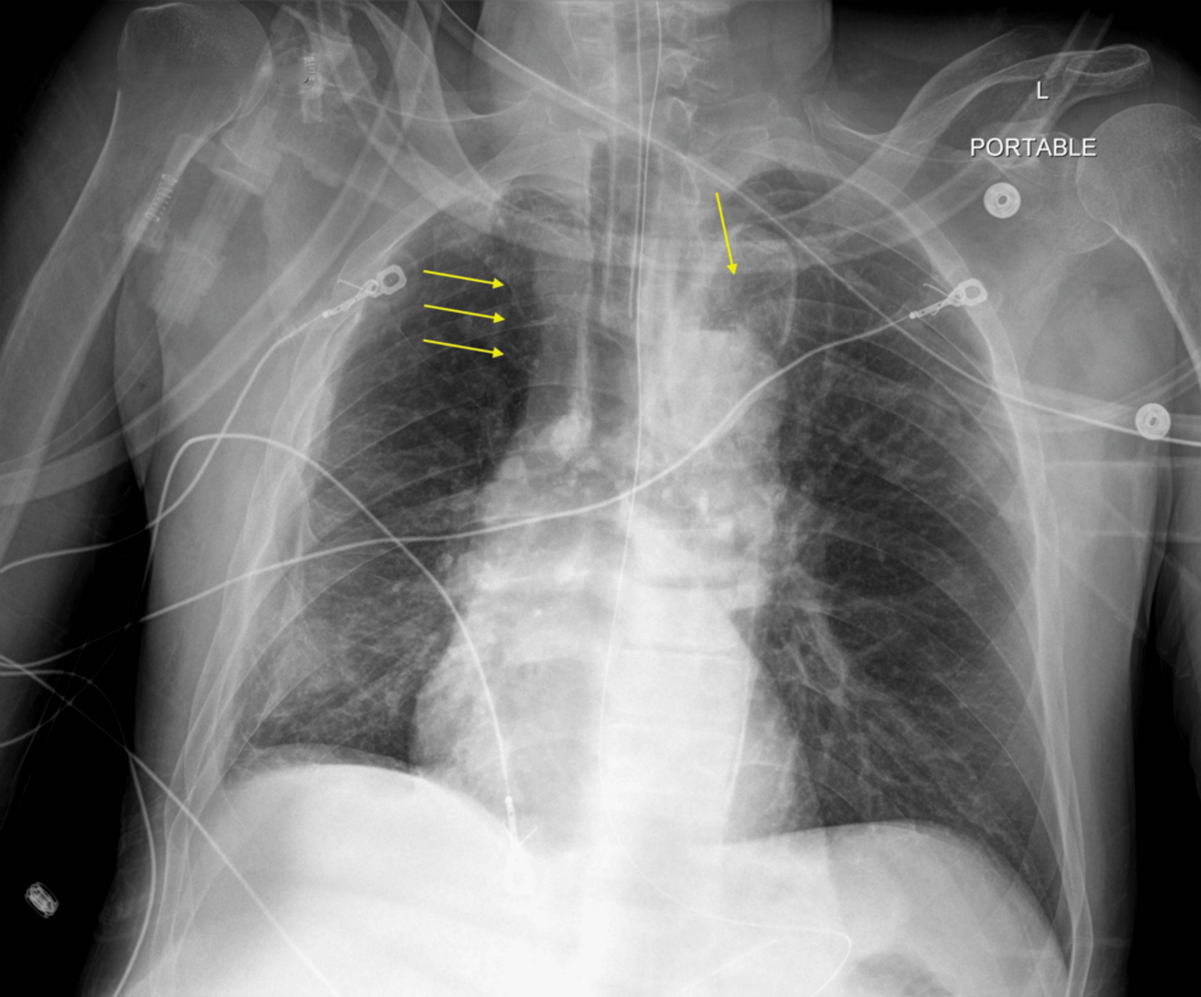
Chest X-ray 12 hours after the event Yellow arrows show expansion of the pneumothoraces
